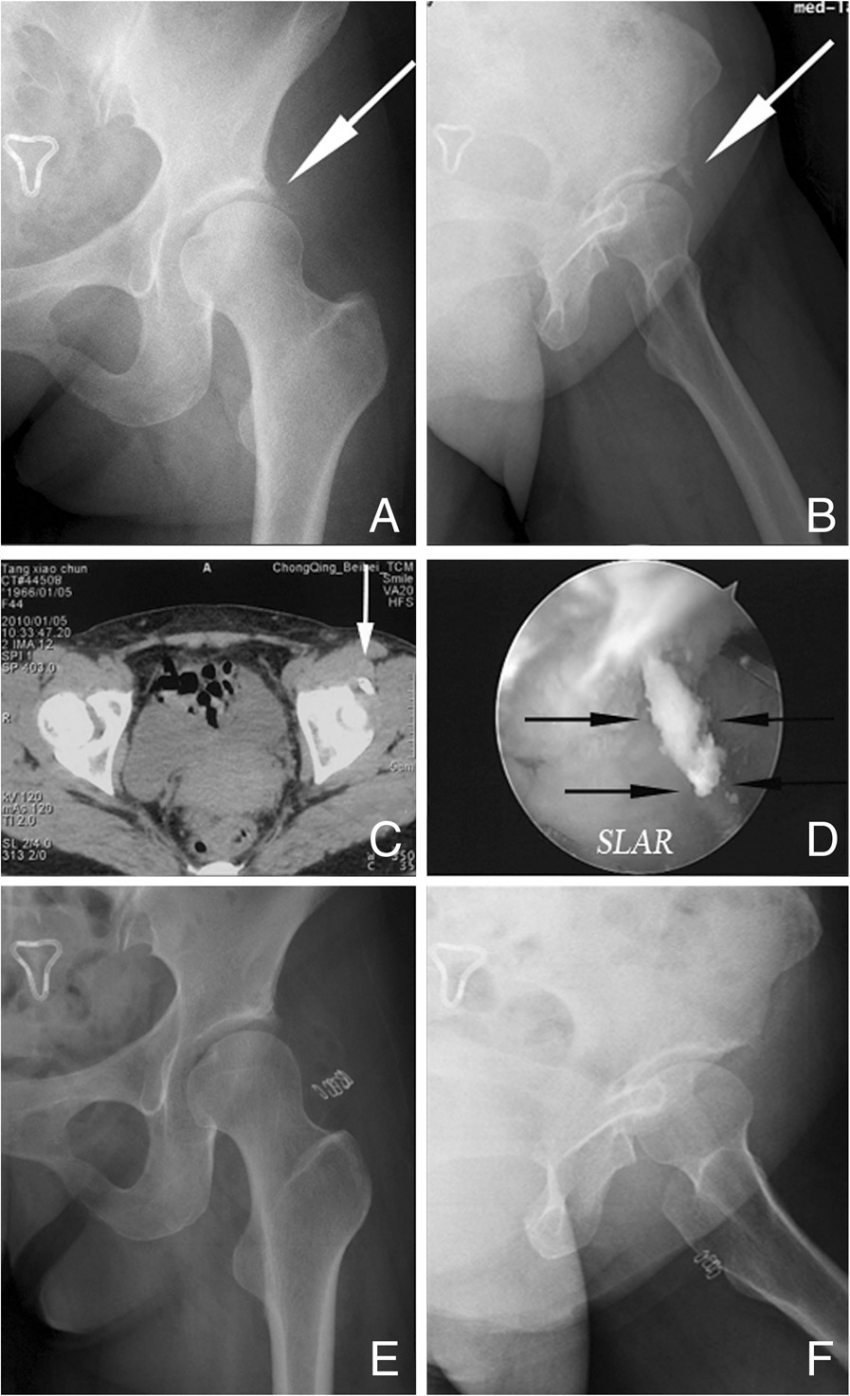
Case 1 radiographic data. (A) The anteroposterior (AP) view and (B) the frog-leg lateral view showed a well-formed triangular radio-opacity (arrow) just adjacent to the prominent acetabular roof. (C) The axial computer tomography slice through the superior part of the hip joint clearly demonstrated the variable calcifications (arrow) in the origin of the rectus femoris. (D) Arthroscopic view of toothpaste-like calcified material (marked with arrows; SLAR, superolateral aspect of the acetabular roof). (E) Postoperative AP and (F) lateral views presented no residual calcium shadow.
